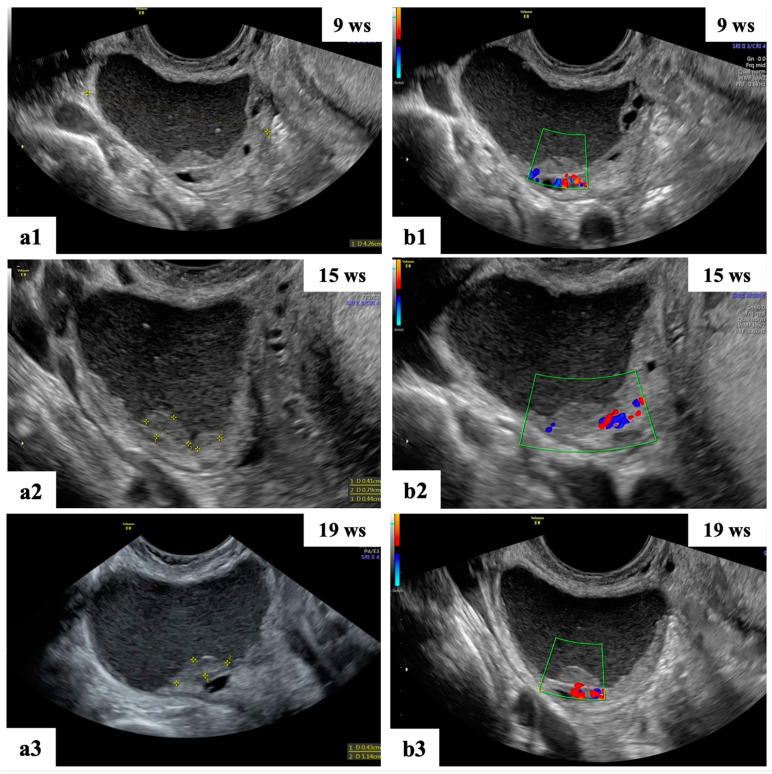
Ultrasound follow-up of the ongoing patient: grayscale (a1–a3,b1) ultrasound images showing unilocular mass with “ground glass” content suspected of decidualized endometrioma. The mass shows papillary projections with regular smooth contours and moderate vascularization at 15 wks (a2,b2) of gestation and reductions in the cyst dimension and number of papillary projections at 19 wks (a3,b3).
